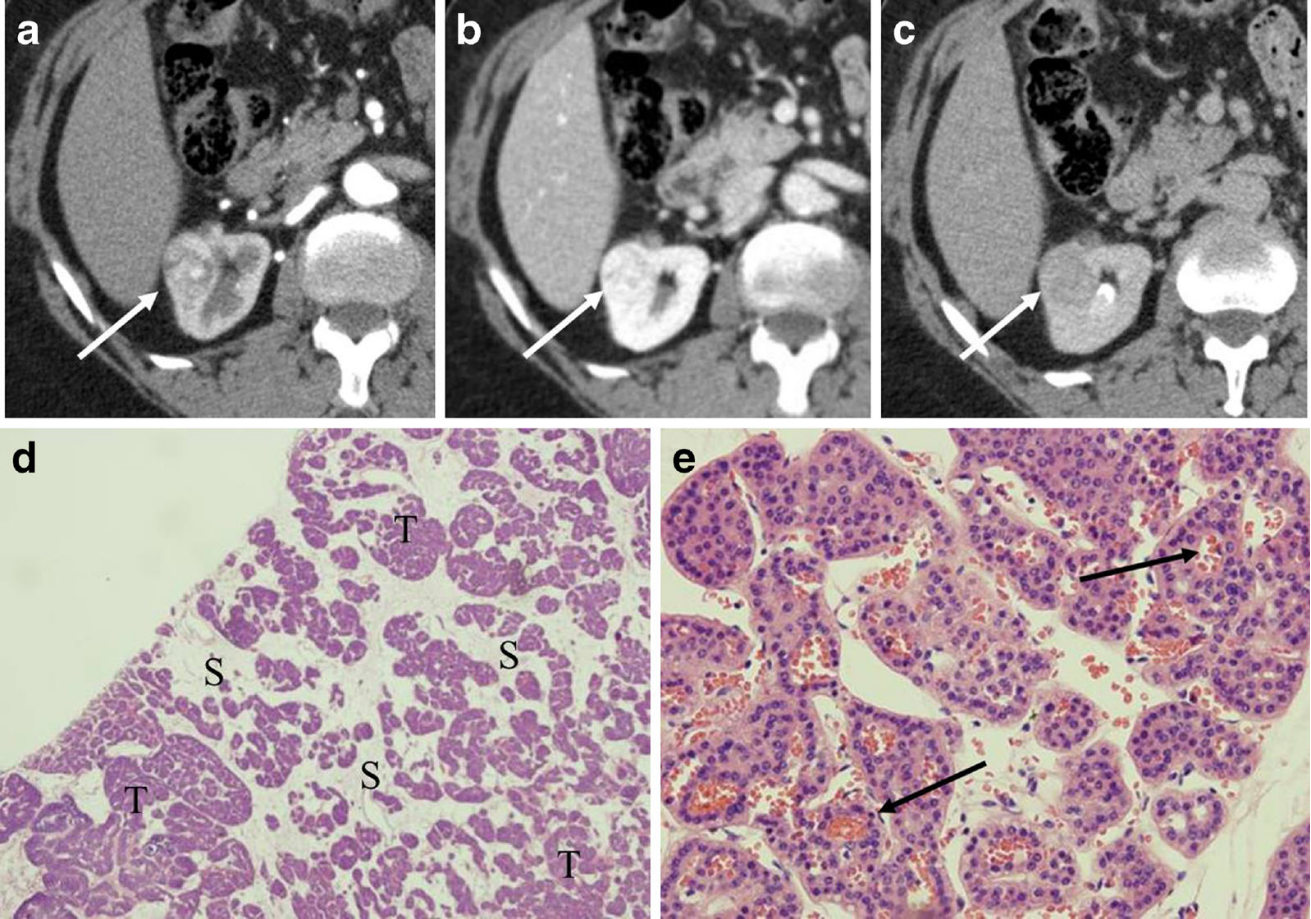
A 72-year-old woman with a right renal oncocytoma demonstrating persistent enhancement in the nephrographic phase. a The arterial phase of the axial CE-CT shows a heterogeneously enhancing mass (*arrow*). b The nephrographic phase shows avid enhancement of the mass (*arrow*). c The delayed phase shows the mass to washout to homogeneously low attenuation (*arrow*). d (Low magnification) Oncocytoma, equal amounts of tumour cells (*T*) and stoma (*S*). e (High magnification) Oncocytoma, organoid pattern with classic polygonal tumour cells with abundant granular eosinophilic cytoplasm, uniformly round nuclei with prominent nucleoli. Abundant small blood vessels are interposed within the stroma (*arrows*)
